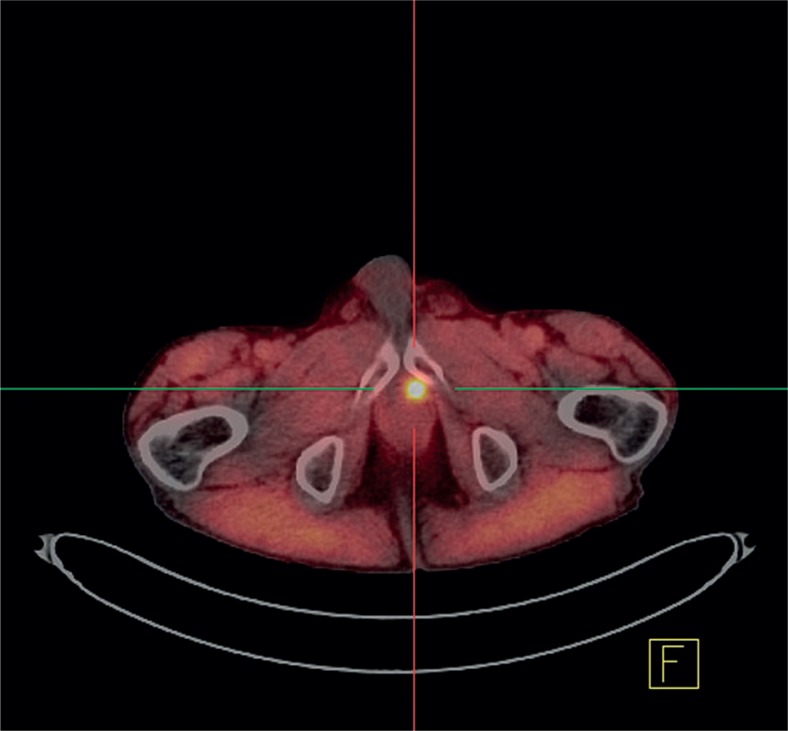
FCH PET/CT: Local recurrence of prostate cancer after radical prostatectomy (Gleason score 8, [PSA] 0.2 ng/ml).
